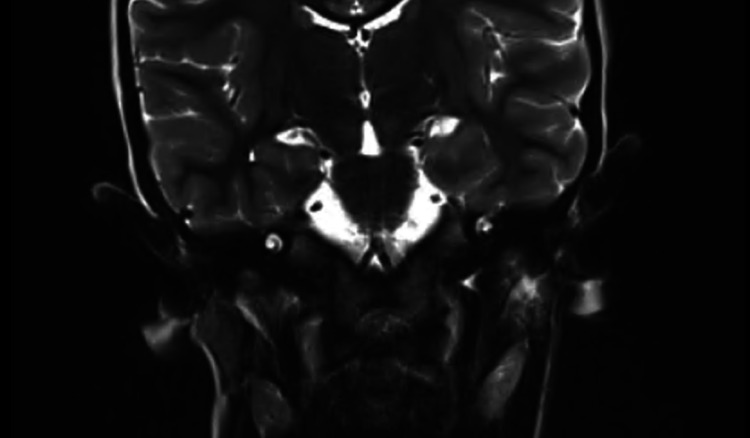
MRI imaging of post-operative recurrence on the left side – coronal view
